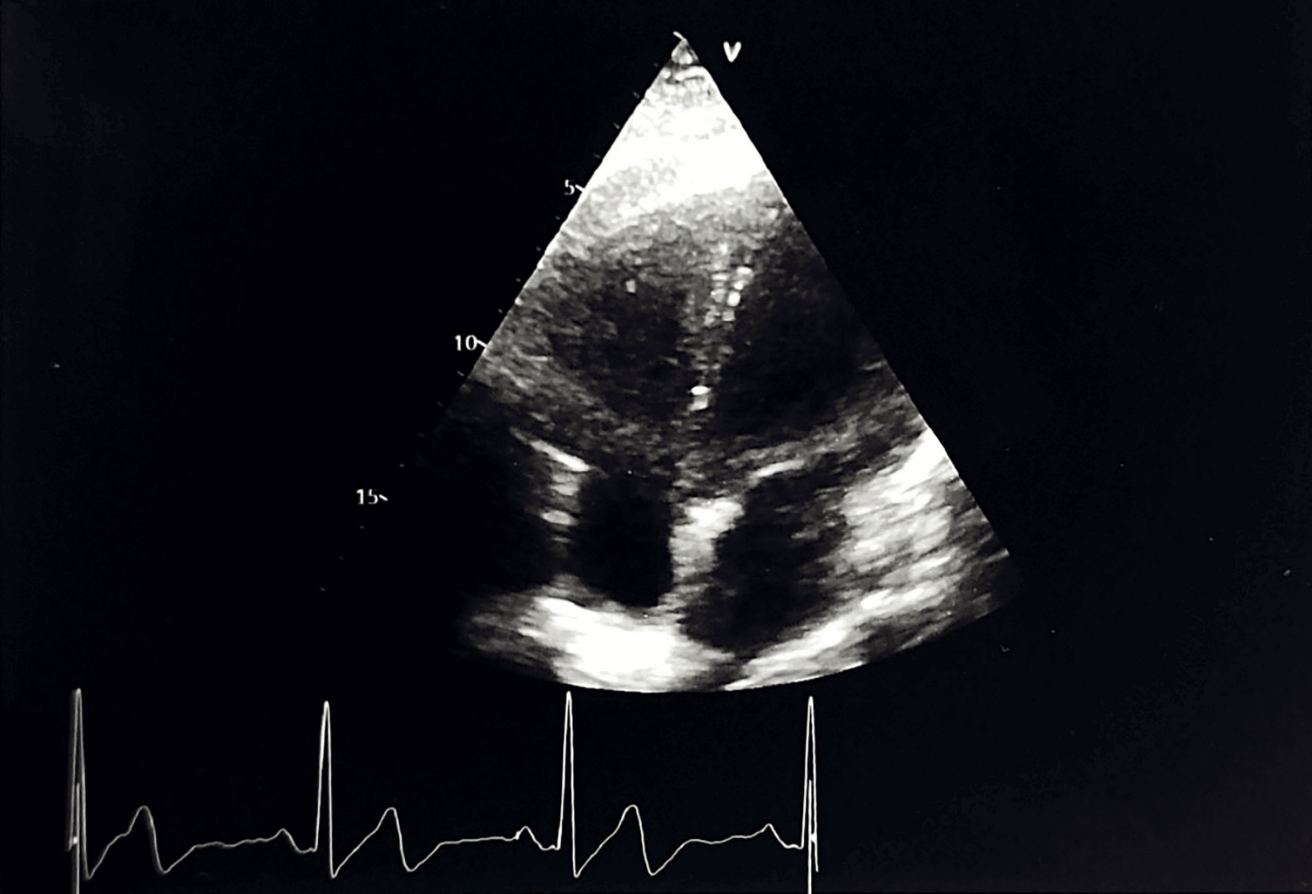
Transthoracic echocardiogram (apical four-chamber view).
